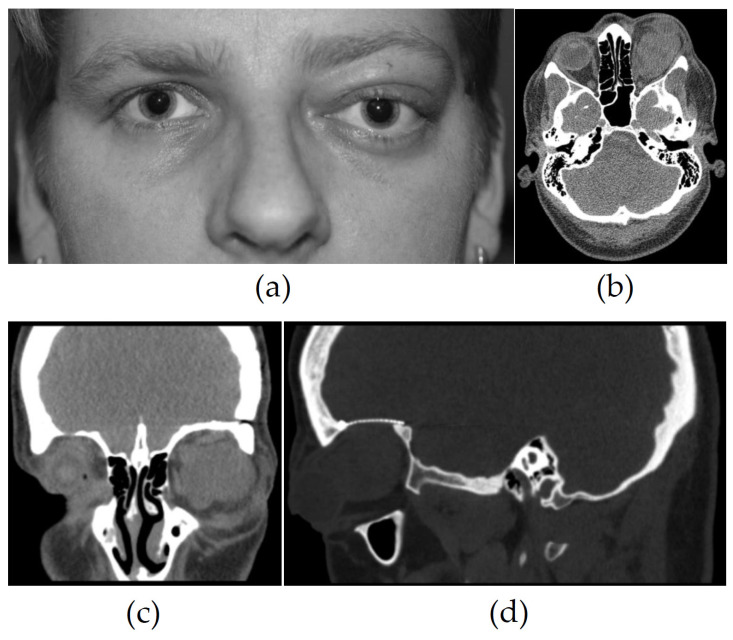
(a) A 33-year-old female patient with painless proptosis of the left eye and no subjective visual impairment. At first presentation, the BCVA was 0.9, IOP 21 mmHg, no history of ophthalmic disease. Computed tomography with contrast media was initially performed due to better availability. (b) Axial, (c) coronary, and (d) sagittal CT revealed a solid hyperdense intraconal mass with compressive growth, with no signs of osseous infiltration. After admission to our neurosurgical department, resection was performed via the transcranial pterional approach. Because of the proximity to the optic nerve, total resection could not be achieved (R1 status). After tumor reduction, the tumor did not show any relevant growth for three years and the patient was followed up regularly in our ophthalmological department. (BCVA: best corrected visual acuity.)
